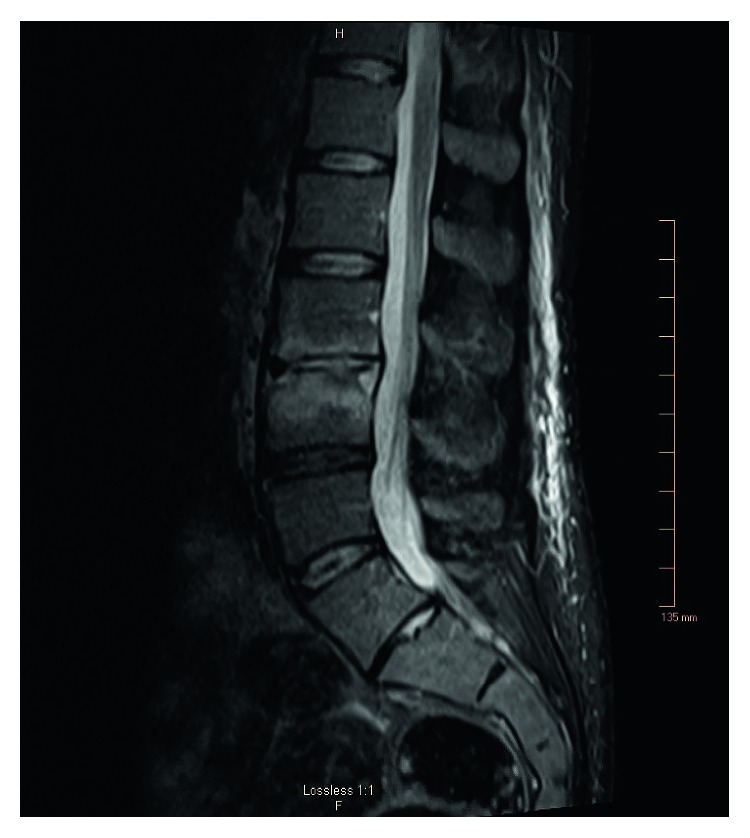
MRI lumbar spine reveals L3-L4 discitis and osteomyelitis.
